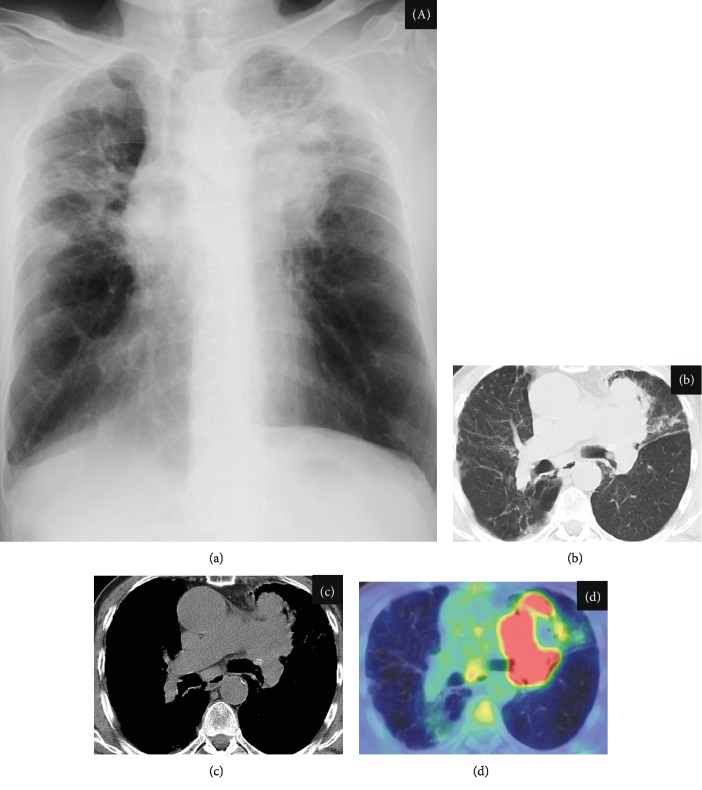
Chest radiography and F-2-deoxy-2-fluoro-D-glucose positron emission tomography/computed tomography (PET/CT) findings. (a) Chest radiography demonstrating a mass-like lesion on the left upper lung field and (b–d) PET/CT showing uptake of FDG by a left pulmonary hilar lesion mass and subcarinal lymph nodes.
